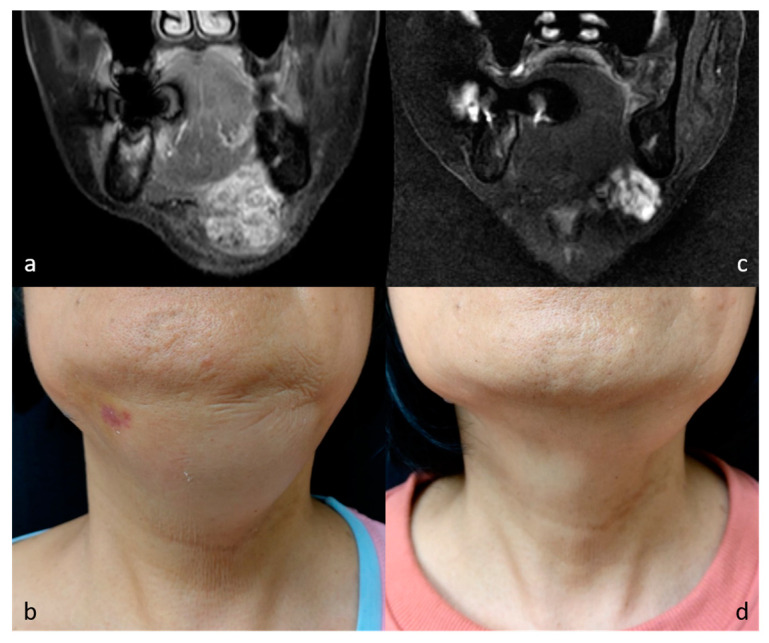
MR images and photographs of patient 4 before (a,b) and after (c,d) RFA (cosmetic scale 4 → 1).
